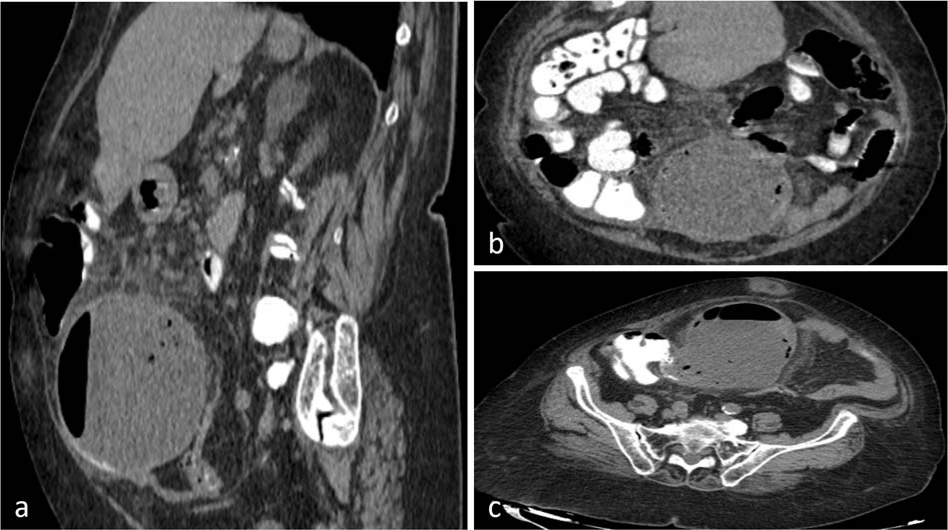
CT scan of the abdomen and pelvis with sagittal (a), coronal (b), axial (c) and views of a well-circumscribed, low attenuation, spherical cystic mass, with internal homogeneous non-enhancing contents and hydro-aerial levels, squalidness of surrounding fat without lymphadenopathy and slightly thickened enhancing wall with mural calcification, located anterior to the right psoas muscle contiguous with the base of the cecum.
